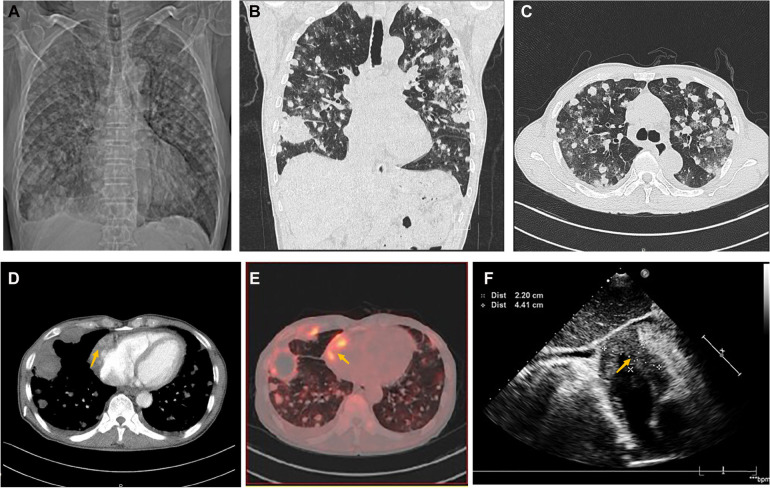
Chest computed tomography demonstrated cardiac mass of Case1. (A) Chest x-ray and Chest CT images in axial (B) and coronal planes (C) showed remarkable extensive pulmonary lesions. (D) Contrast-enhanced CT showed a hypodense oval filling contrast defect (arrow) at the right atrium (RA). (E) 18F-fluorodeoxyglucose positron emission tomography/CT (18F-FDG PET/CT) showed more uptake in the RA mass (arrow), lung, and pleura. (F) Echocardiography depicted an oval-shaped mass (22 × 41 mm) (arrow) in the RA at the level of the superior vena cava ostium.
